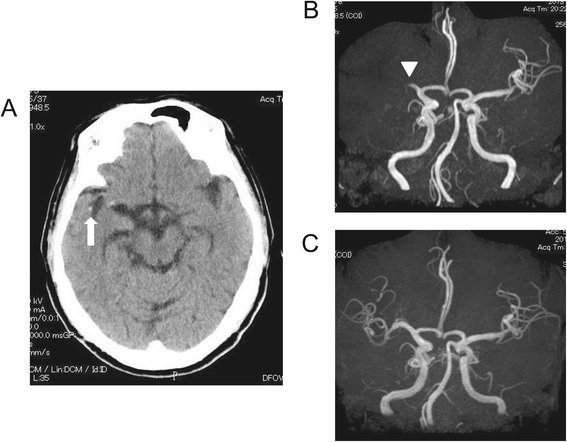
Brain images after the onset of cerebral infarction. A: Noncontrast brain computed tomography revealed a “dot” sign in the right middle cerebral artery (MCA; arrow) seen as a dot in the sylvian fissure. B: Brain magnetic resonance angiography (MRA) showing an occlusion of the right MCA (arrowhead) on the day of the stroke. C: Brain MRA 3 days after the stroke showing blood flow of the damaged area of the brain.
